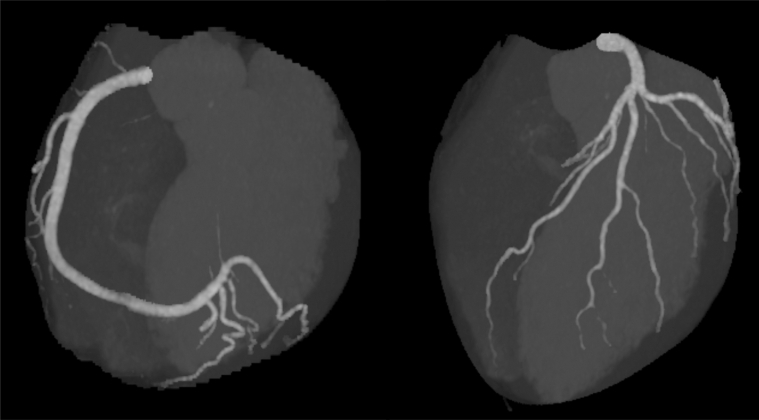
Coronary CT Angiography in the 35-Year-Old Son Coronary CT angiography demonstrating normal coronary anatomy without stenosis or plaque and a calcium score of 0. CT = computed tomography.
